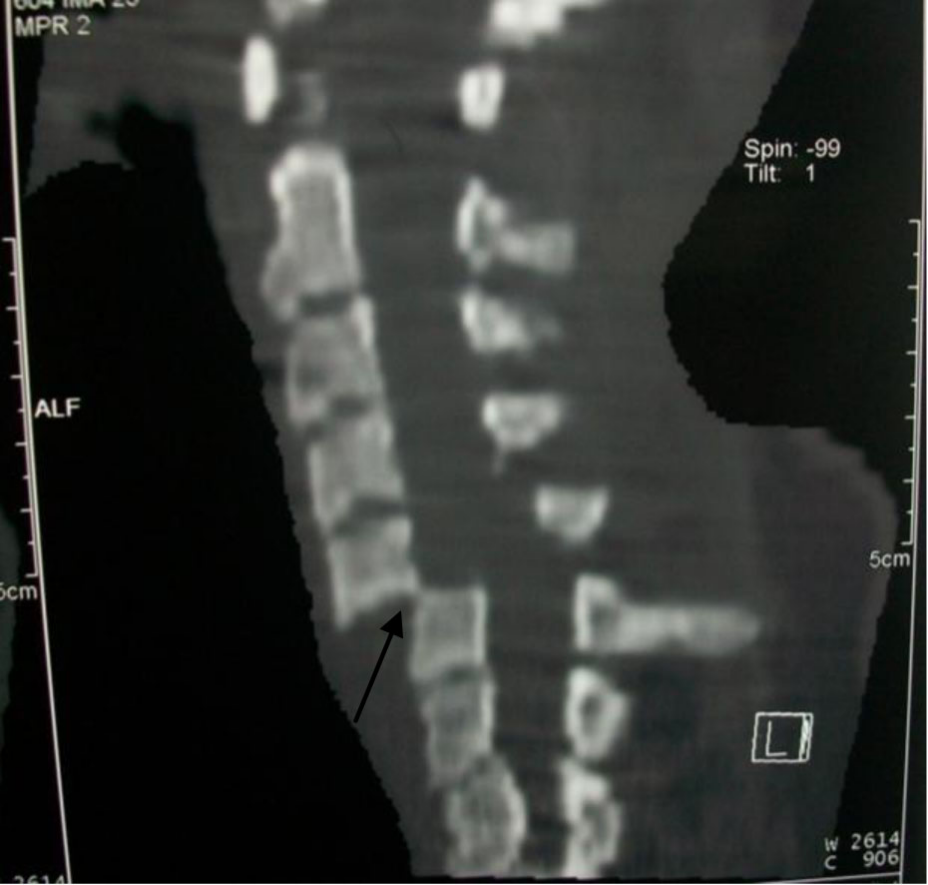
CT scan of the cervical spine revealing complete anterior sublaxation (C5 over C6 cervical vertebrae).
